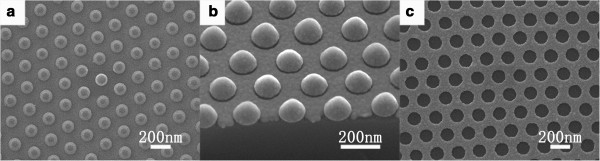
SEM images describing the formation of the porous Ag film template. (a) SEM image of the sample after RIE treatment of 55 s. (b) SEM image of the sample after 5-min Ag deposition. (c) The sample after removal of the PS spheres by ultrasonication.
